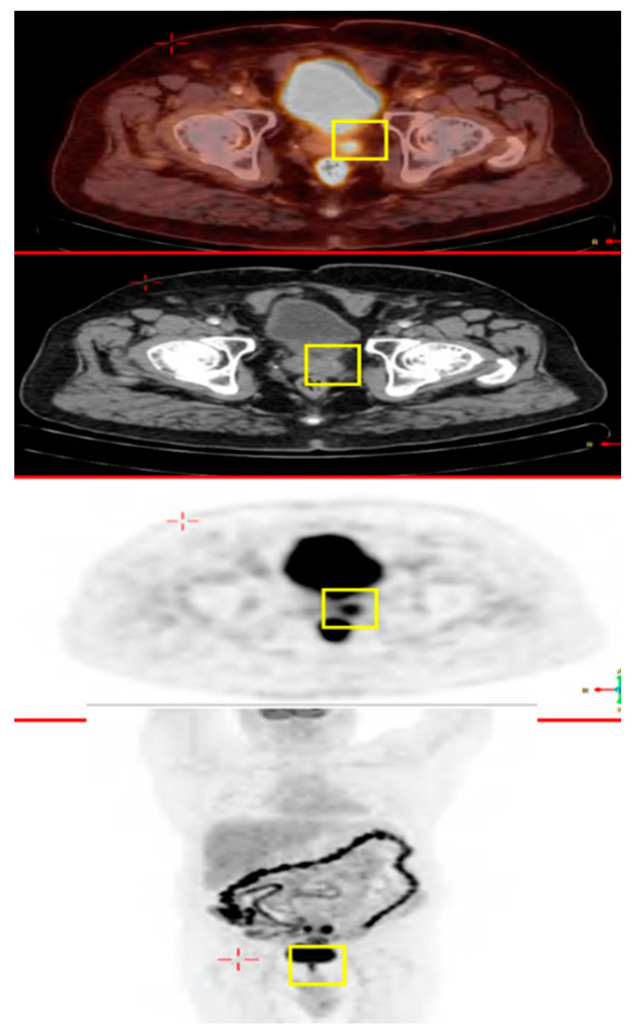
Images from the patient’s PET-CT showing the hyper-metabolic uptake prostate in the area of the (yellow squares).
